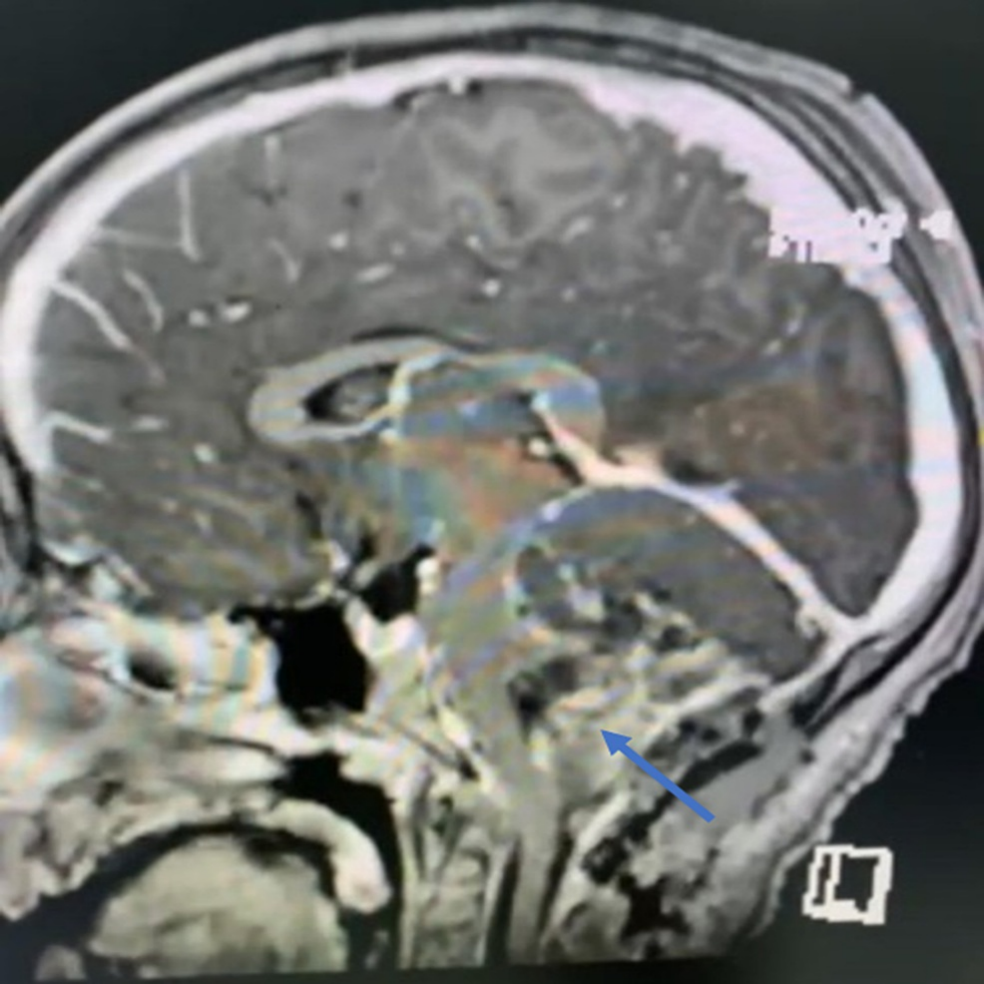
Postoperative image - MRI brain sagittal cut
